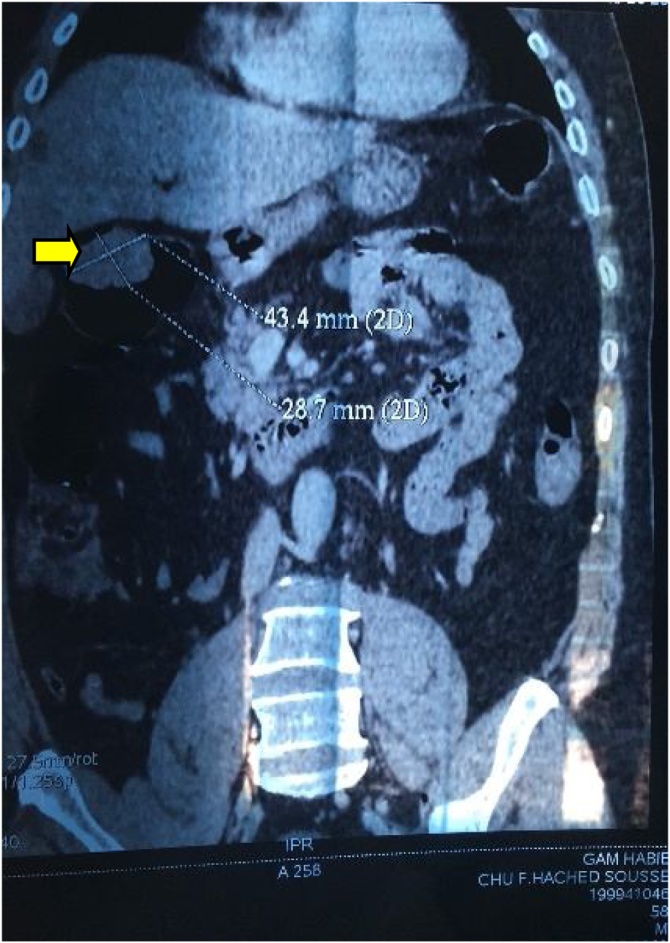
Abdominal CT scan showing a polypoid mass (⇆) in the right colon.
